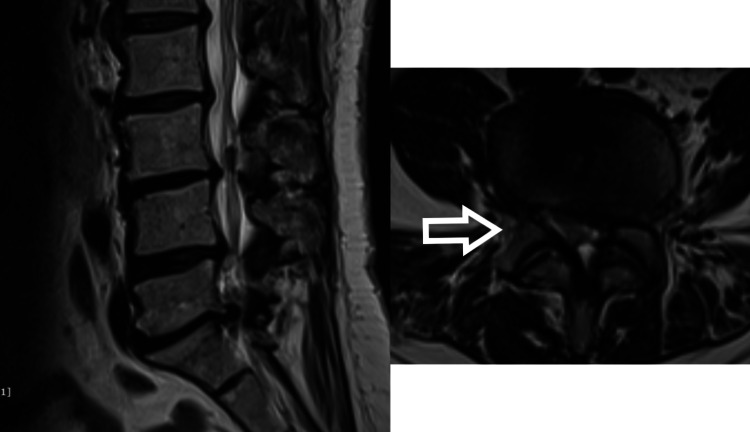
Pre-operative MRI showing Lumbar canal stenosis responsible for claudication symptoms
